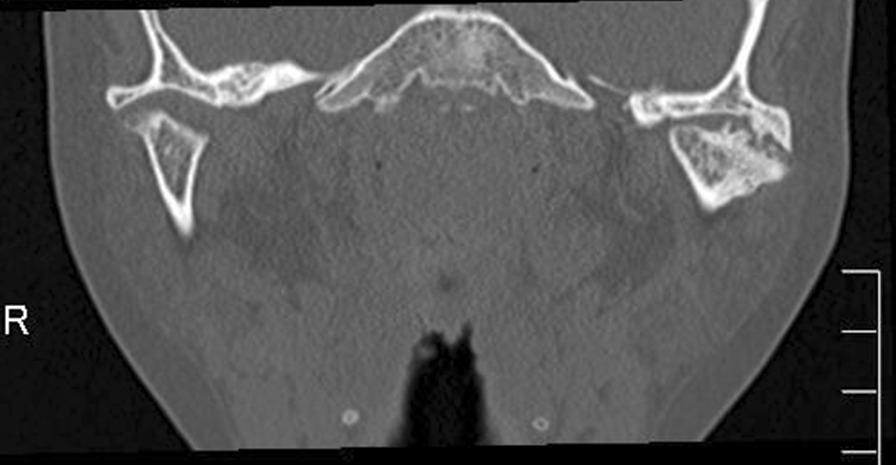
Preoperative computed tomography demonstrated that the mandibular condyle and glenoid fossa were almost osteoadhesive
